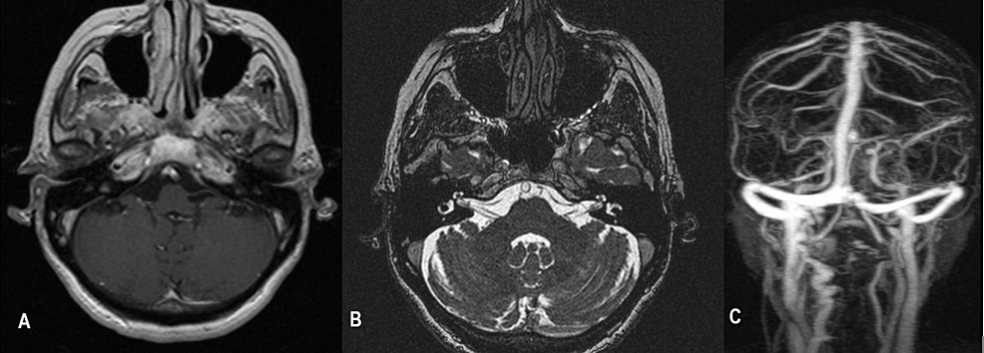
(A) Postcontrast axial T1WI MRI (B) Postcontrast axial T2WI MRI and (C) MRA scan of the circle of Willis excluded any identifiable cause of the unilateral hearing loss.
